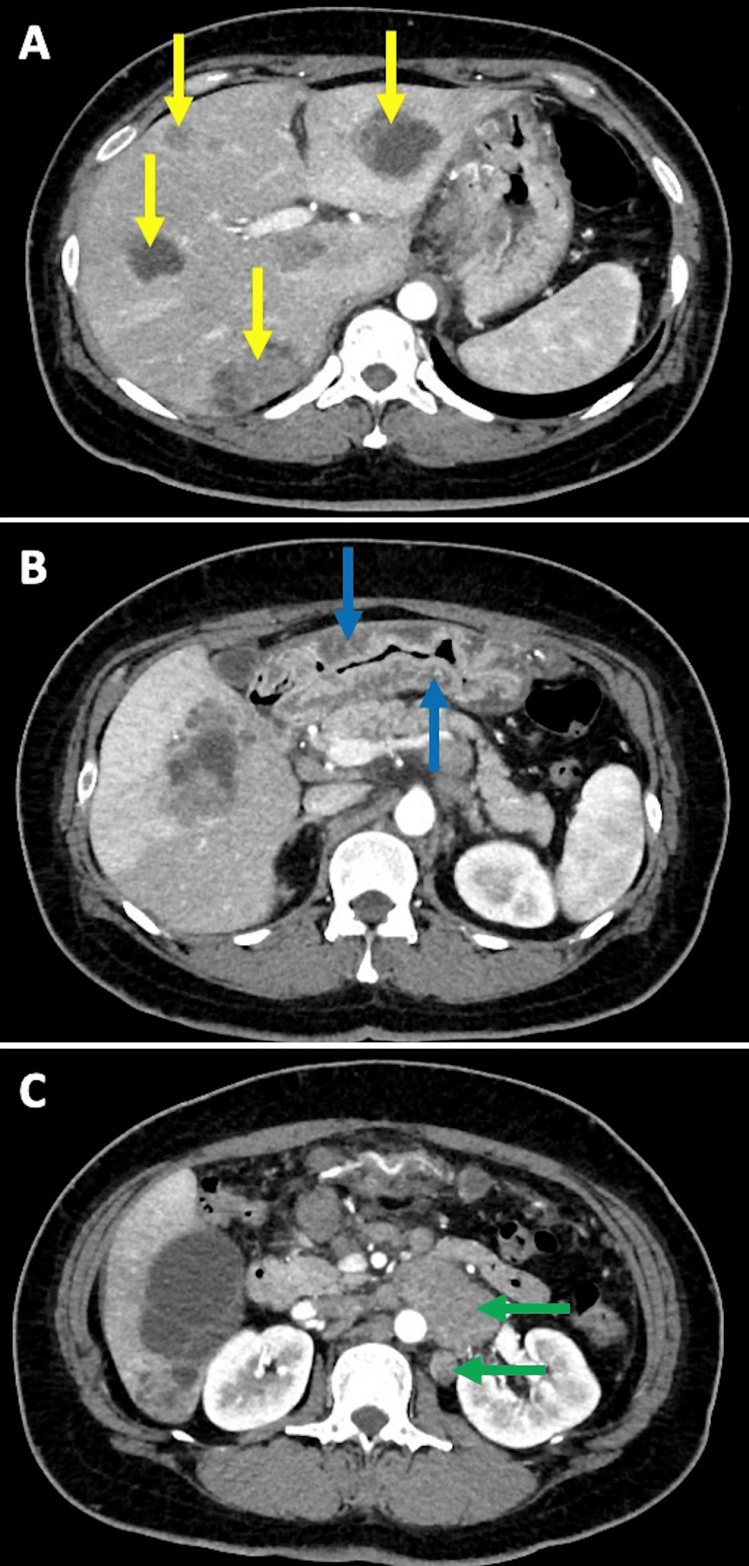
Contrast-enhanced computed tomography showed (A) multiple tumors with mixed cystic and solid components in the liver (yellow arrows), (B) gastric wall thickening (blue arrows), and (C) multiple enlarged lymph nodes (green arrows).
